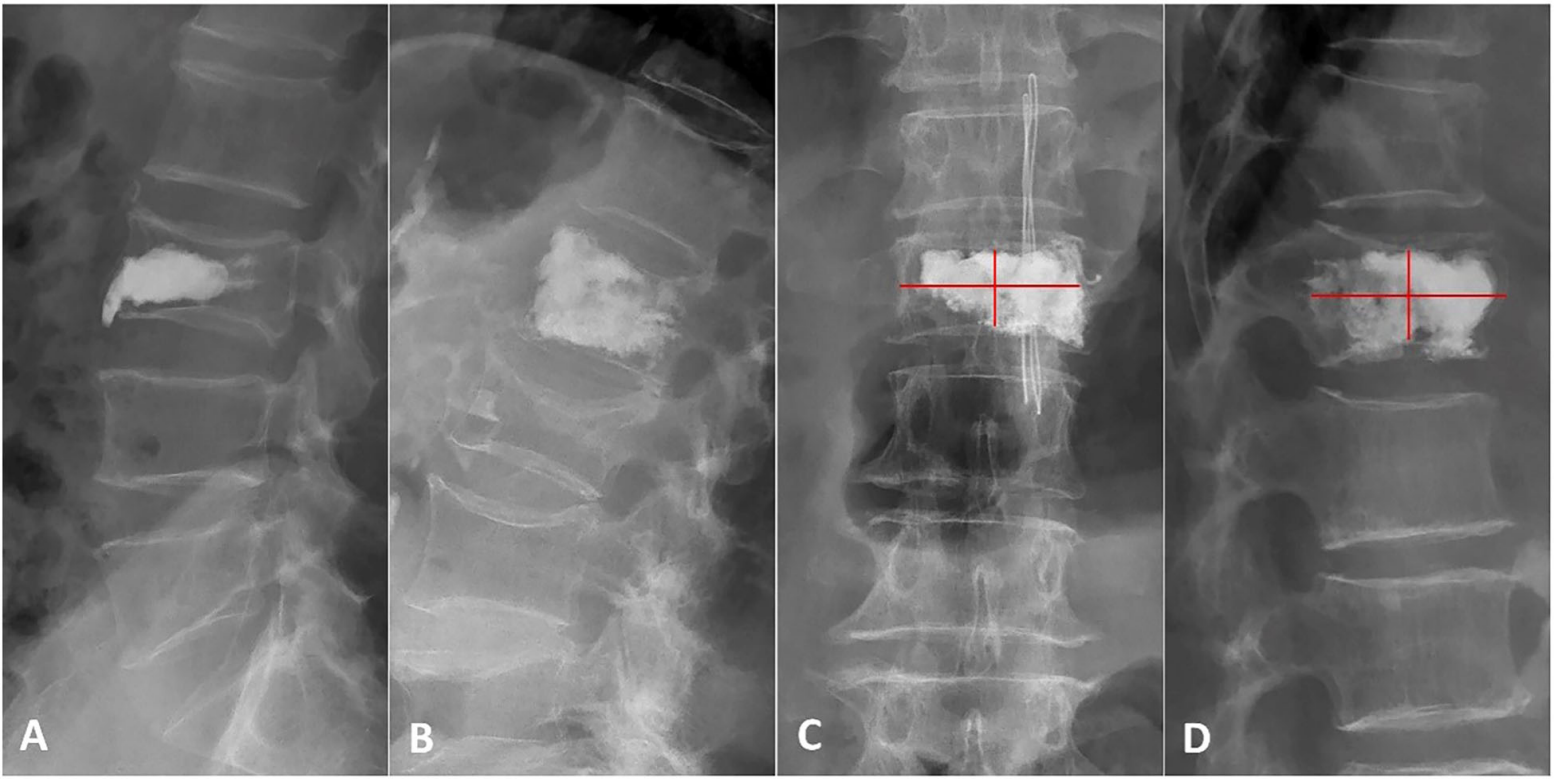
Patterns and scores of bone cement distribution. (A) Lateral X-ray showing a blocky cement pattern. (B) Lateral X-ray showing a spongy pattern. (C, D) Anteroposterior and lateral X-ray using the 12-score method: the vertebra is divided into four quadrants. 2 scores are counted if the bone cement filling exceeds one-third of each quadrant, bone cement contacted the upper or lower endplate or bone cement crossed the middle line.
